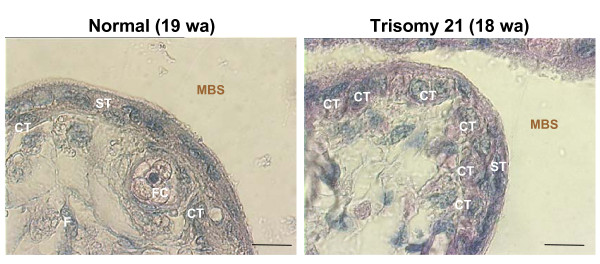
Second trimester chorionic villi of normal (19 weeks of amenorrhea: wa) and trisomy 21 (18 wa) placentae. In normal placenta, a large amount of cytotrophoblastic cells (CT) have fused into a thin multinucleated syncytiotrophoblast (ST). In trisomy 21 placenta, many cuboidal cytotrophoblastic cells (CT) are still present beneath the syncytiotrophoblast (ST) increasing the thickness of the trophoblastic layer. Scale bar = 10 μm.
